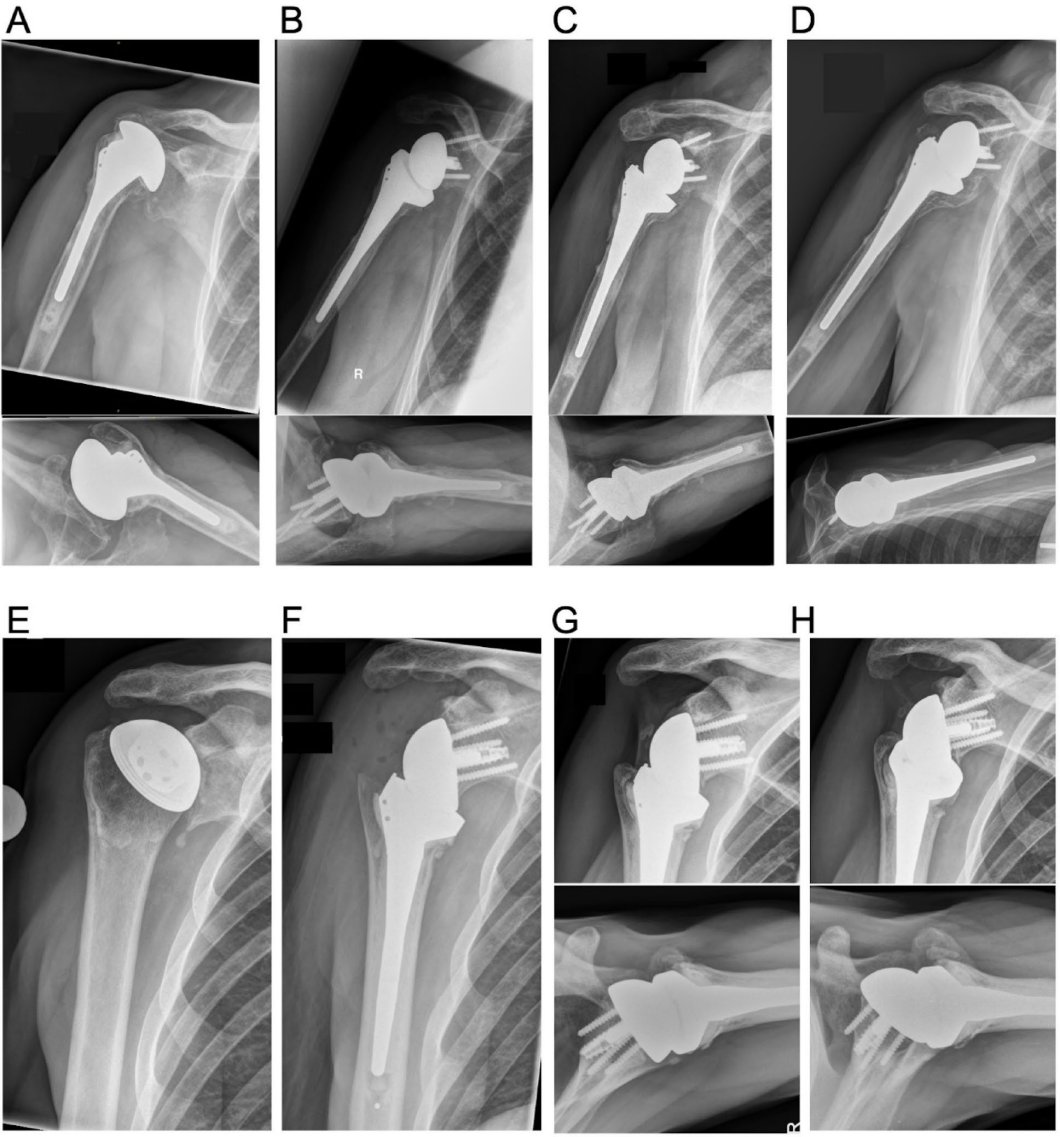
A Preoperative true anteroposterior (a. p.) and axial view of the right shoulder of a 62-year-old patient with a cemented stemmed hemiarthroplasty with severe glenoid erosion and massive cranialization of the humeral head due to secondary mass defect of the rotator cuff. B Postoperative true a. p. and axial view after one-stage revision to RSA with a cemented stem. C 14 months postoperative: Beginning calcification at the medial humeral metaphysis and the inferior scapular neck. D Manifest ankylosis due to fusing Heterotopic Ossification at the long-term follow-up at 12 years postoperatively, a Y-view was taken as the axial view was no longer possible. E Right shoulder of a 64-year-old male patient with glenoid erosion with a stemless hemiarthroplasty. F Postoperative view of the same patient after revision to a cemented standard-stem RSA. G Formation of small bony islands in the lateral capsule at 18 months postoperative. H The radiographs 9 years postoperatively show a stable course of the small bony islands.
